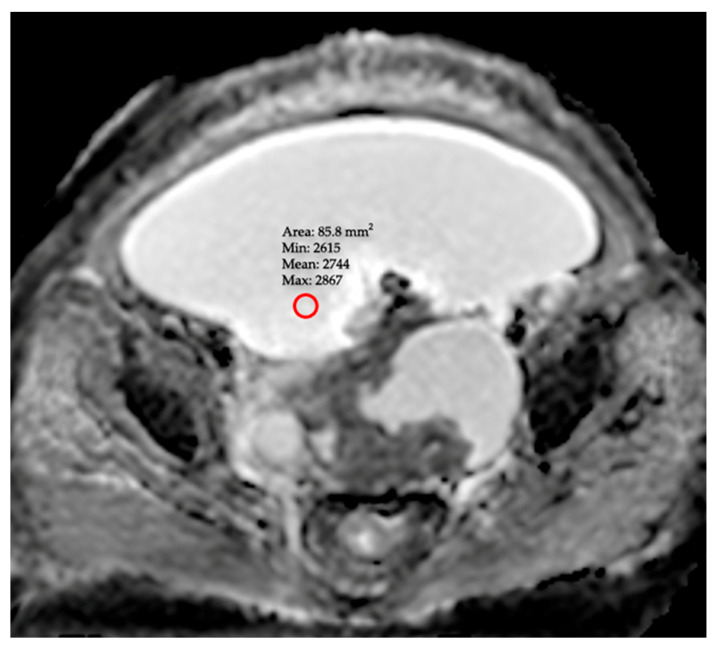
DWI MRI image in a patient with ovarian cancer, demonstrating a ROI placed within the intra-abdominal free fluid for ADC measurement (red circle).
